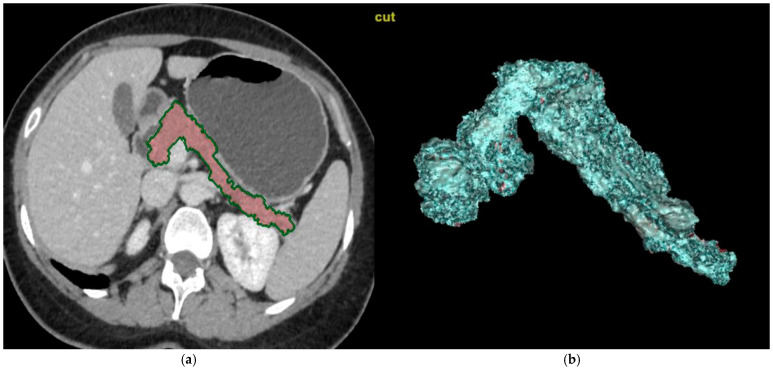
Calculations of the pancreatic volume on CT images. (a) The CT scans were transferred to workstations manufactured by GE in the USA, model AW Volume Share 7. Using manually controlled cursors, radiologists outlined the outer boundary of the pancreas during the parenchymal phase on axial images. Fatty tissue and vascular structures, such as the splenic artery and the portal vein, were excluded to the greatest extent possible. (b) The total volume of the pancreas was calculated using customized three-dimensional volumetric software (AW4.7 Ext.13 Software, GE, Chicago, IL, USA) on workstations within the designated area.
